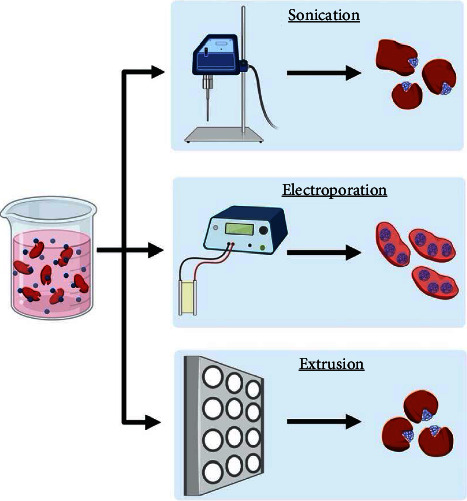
Platelet and thrombolytic therapeutic fusion techniques. Platelets can be loaded with thrombolytics through sonication, electroporation, or extrusion. Sonication uses ultrasonic waves to fuse cargo and membranes together [44], while electroporation exposes membranes to electrical waves, creating pores through which cargo can enter [45]. Extrusion requires both cargo and membranes to be run through a porous membrane, where mechanical pressure forces them to interact [46].
